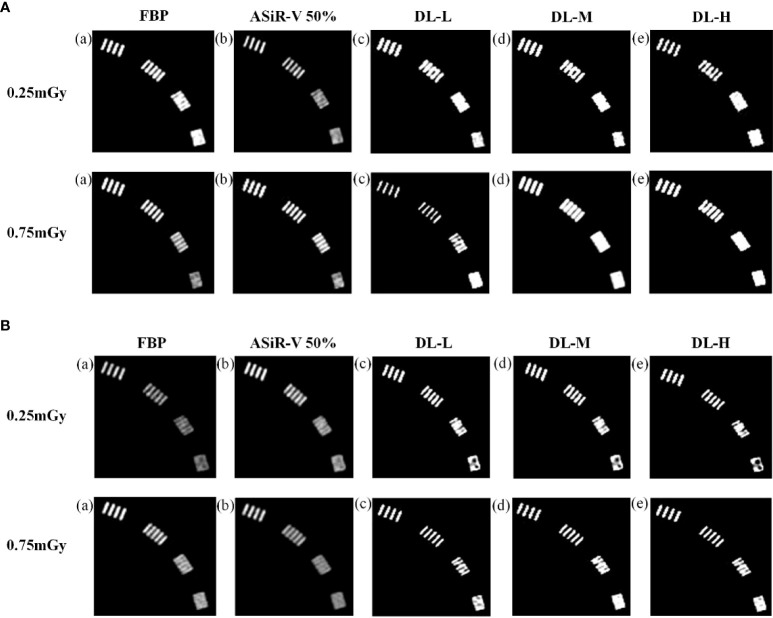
High-contrast images in helical mode reconstructed with FBP (a, f), ASiR-50% (b, g), and DLIR (L/M/H) (c, h; d, i; e, j) at 0.25mGy and 0.75mGy with a slice thickness of 1.25mm (A) and 5mm (B), respectively. CT, computed tomography; FBP, filtered back projection; ASiR-50%, adaptive statistical iterative reconstruction-veo 50%; DLIR(L/M/H), deep-learning image reconstruction, level low, medium, and high; mGy, milligray.
